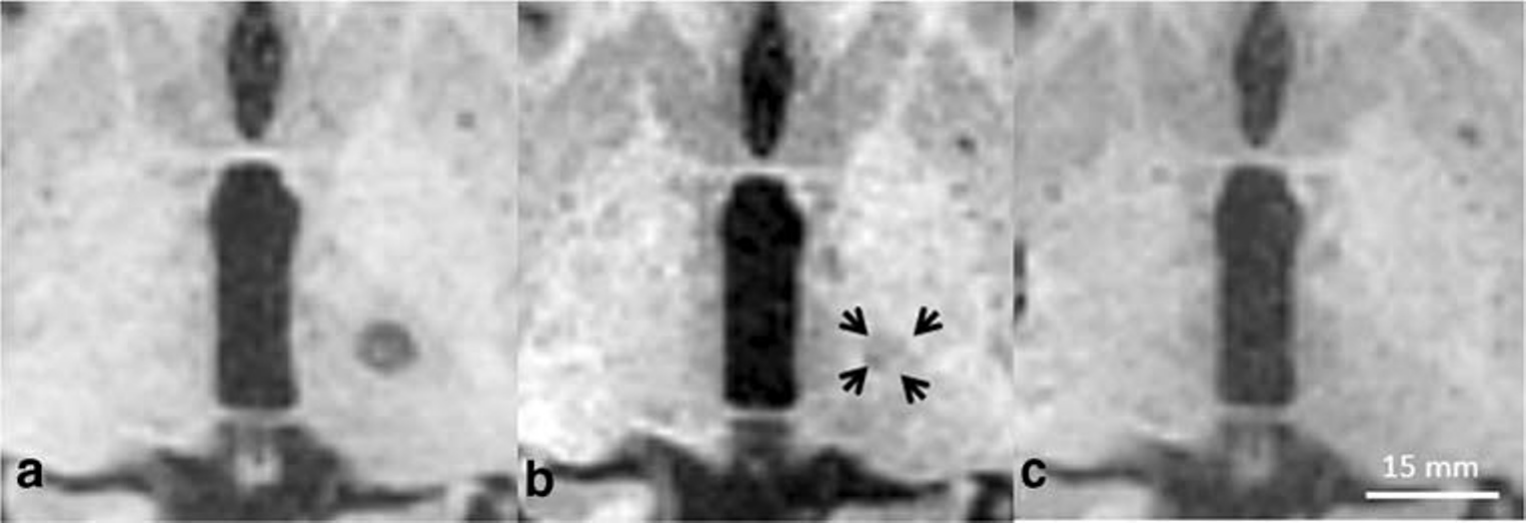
Fading of the MRgFUS lesion on T1-weighted images. While on SWI and T2-weighted images shrinkage of the lesion was noted, the lesion rather faded in signal intensity compared with the surrounding brain tissue. a Day 3, b day 30 (black arrows indicating lesion margins) and c “vanished” lesion on day 180
